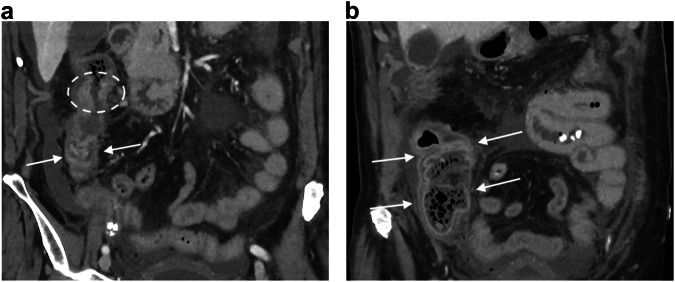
Ischemic colitis complicating a right colonic cancer. Axial and coronal portal phase CT (a, b) in an 82-year-old woman shows a stratified enhanced thickening (arrows) upstream a short and irregular unstratified thickening (dotted circle) of the right colonic wall
